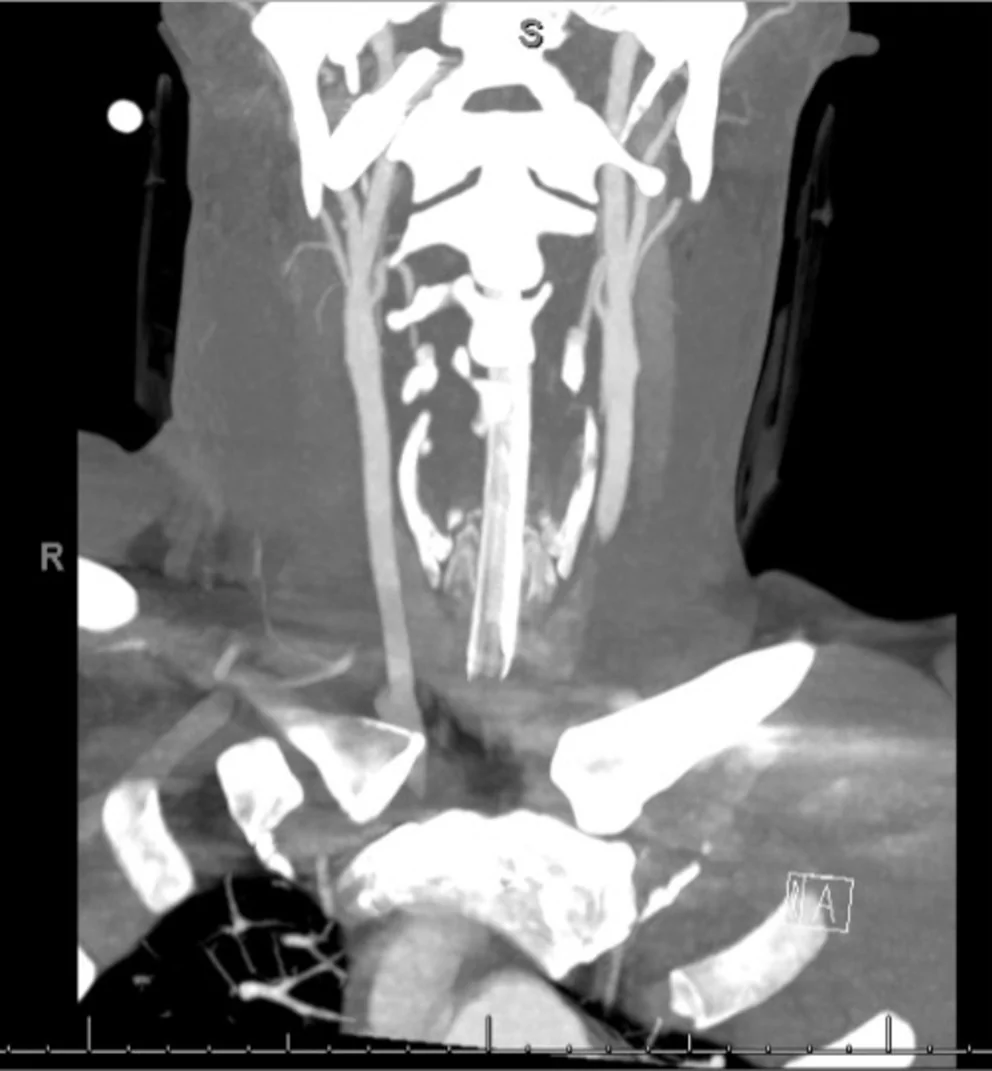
Coronal image of CTA neck showing vape pen touching the carotid artery wall.
